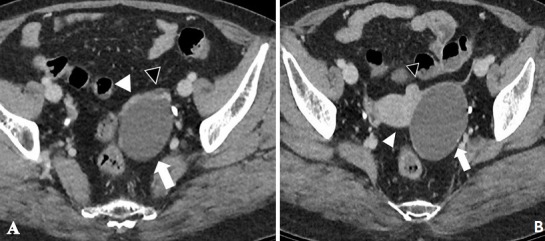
(A) contrast-enhanced CT images show a unilocular cystic lesion (white arrow) abutting the left ovary (black arrowhead) and fallopian tube (white arrowhead); (B) contrast-enhanced CT images show a unilocular cystic lesion (white arrow), uterus (white arrowhead) and myoma (black arrowhead)
